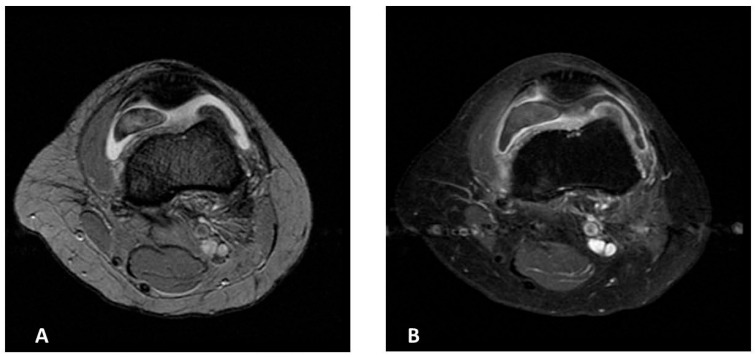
MR images of the left knee (A,B). (A) Axial T2-weighted MR image of the suprapatellar pouch of the left knee showing intraarticular lesion with cloud-like dark areas due to hemosiderin deposition. (B) Post-contrast T1 fat-saturated MR image of the same region demonstrating synovitis and enhancement of synovial proliferations.
